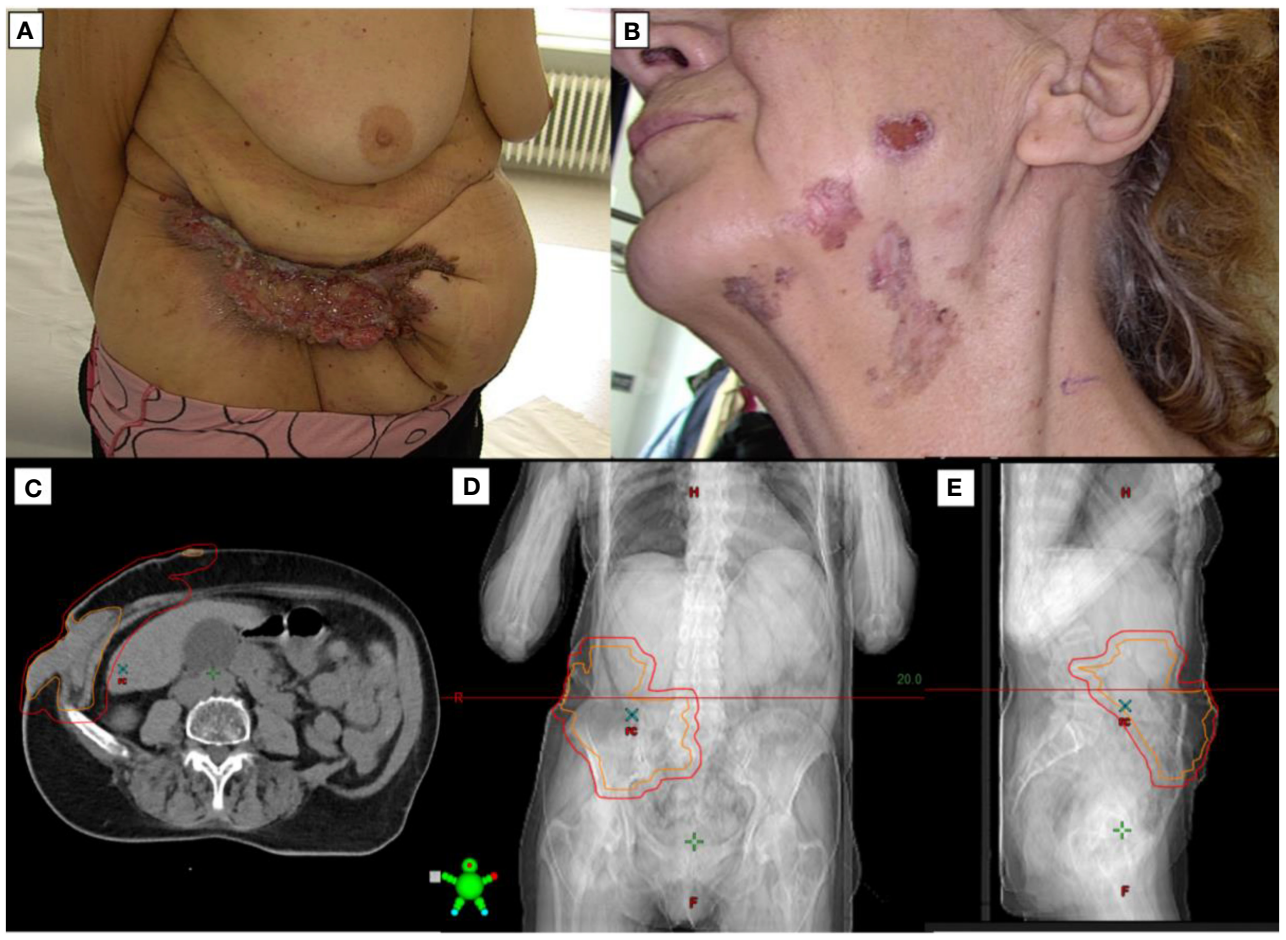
Overview of a patient with giant BCC before treatment. (A) An 68-year-old woman presented with an enormous, fetid, abdominal wall mass. (B) Lesions of BCC on the face and neck. (C) Axial CT of the tumor, (D) Coronal CT of the tumor, (E) Sagittal CT of the tumor.
